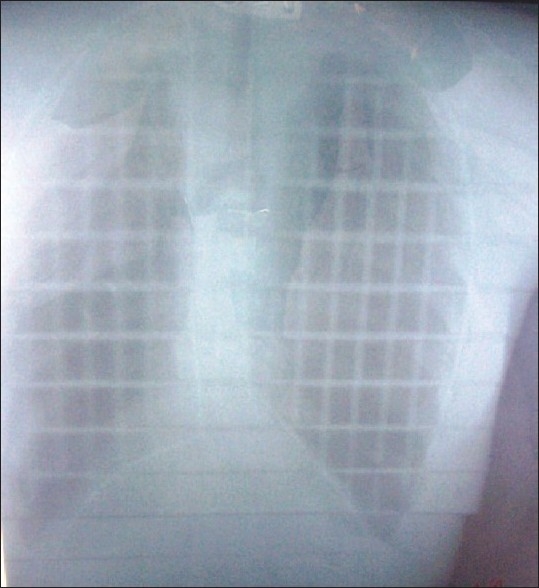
Verification Radiograph with Lead Wire Positioned around Periphery of Styrofoam Shield Template
